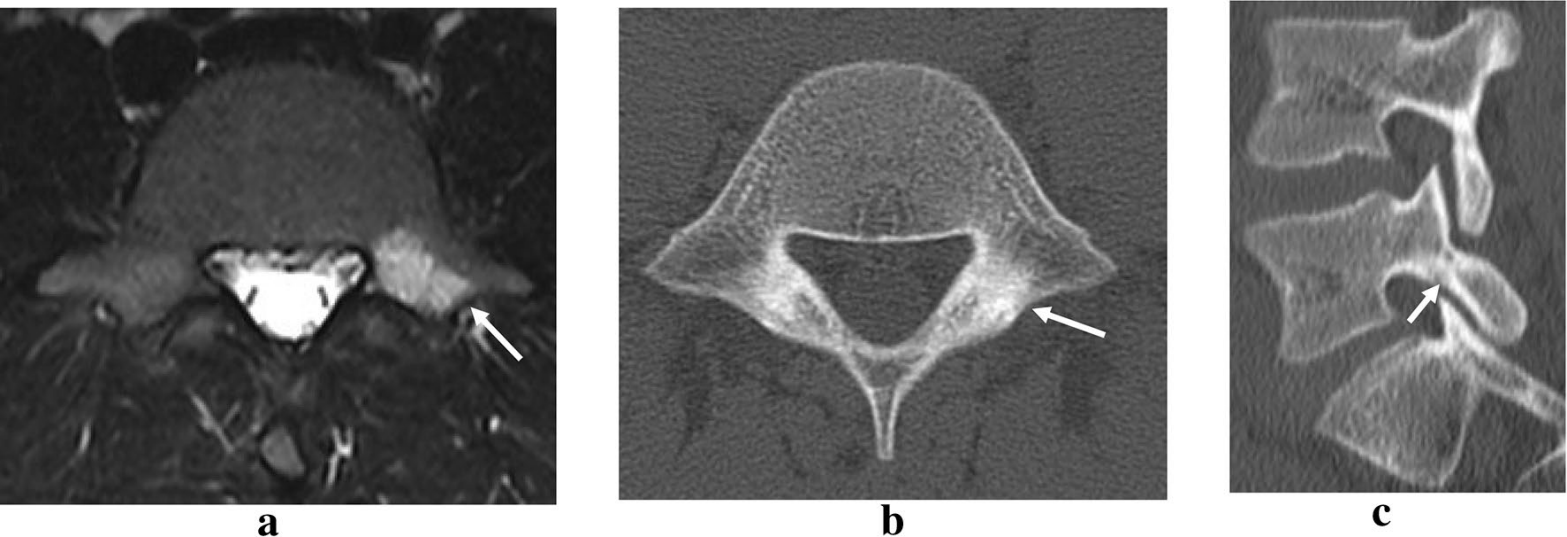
Twelve weeks after the first healing, short tau inversion recovery magnetic resonance images (STIR-MRI) showed high intensity change (white arrow) on only the left side (a). CT revealed no fracture line (white arrow) in the axial view (b) but slight bone resorption (white arrow) on the ventral side in the sagittal view (c).
